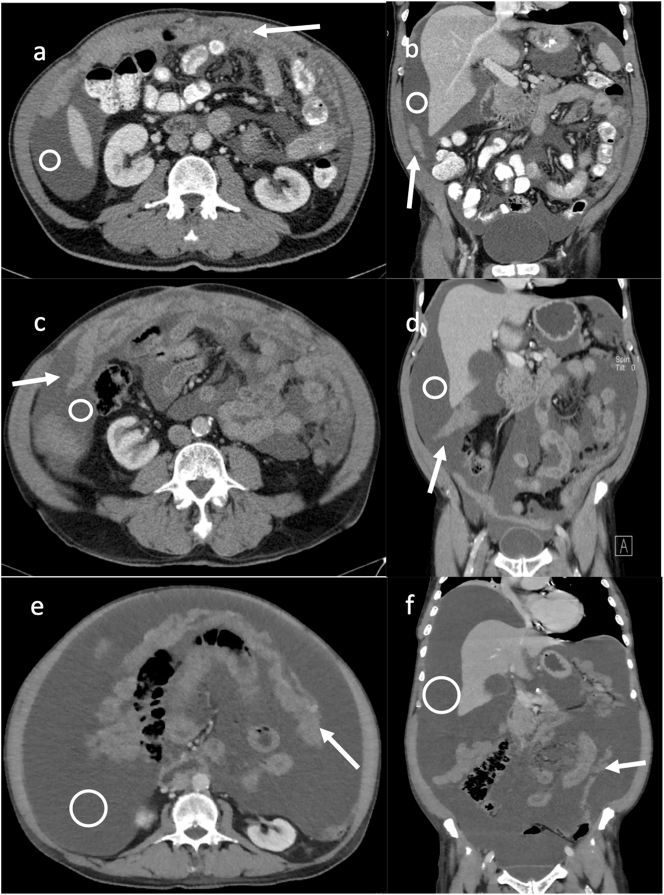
Represented are the contrast enhanced staging CT scans of the patient at three different occasions in axial and coronal slices depicting the ascites (white circle) as well es the omental cake (white arrow) as surrogate for the extent of the disease. (a) and (b) initial CT scan May 2017, (c) and (d) May 2022, (e) and (f) January 2024.
